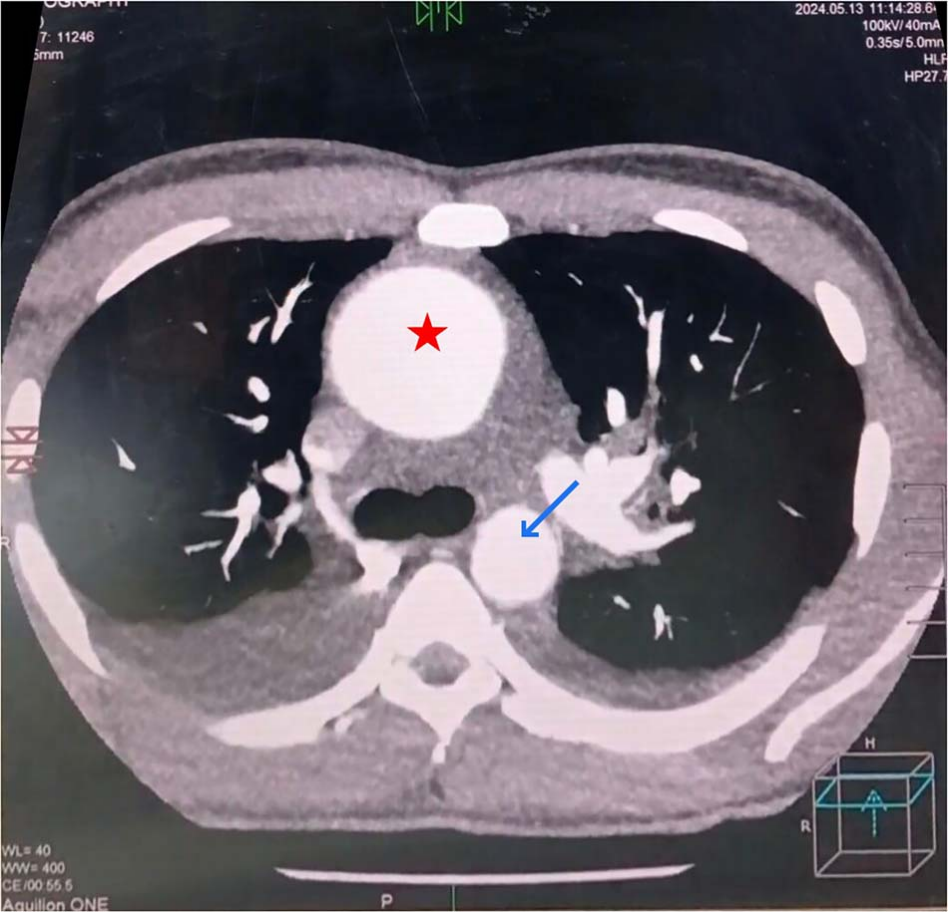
Transverse section of CT aortography showing the massively dilated ascending aorta (star) and descending aorta (arrow).
